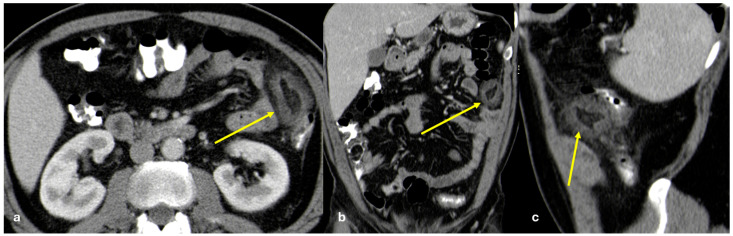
Epiploic appendagitis. A middle-aged man with abdominal pain mainly located on the left upper quadrant. Portal venous phase CT shows a fat-density, round, and small lesion surrounded by a high-attenuation rim (hyperattenuating ring sign) abutting anteriorly the descending colon ((a): axial, (b): coronal, and (c): sagittal images; arrows).
